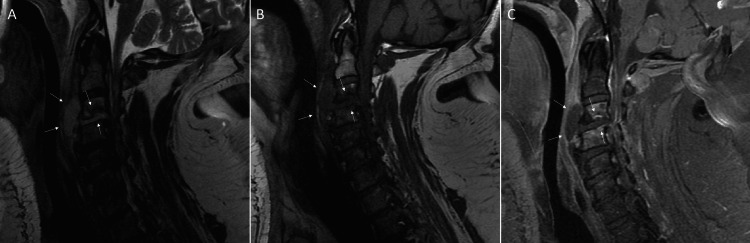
MRI of the cervical spine with (A) fat saturated T2 imaging, (B) T1 imaging, and (C) T1 imaging with gadolinium-based contrast showing evidence of discitis/osteomyelitis centered at C3-4 with adjacent paravertebral abscesses, and suggestion of epidural phlegmon in the anterior epidural soft tissues of C2 and C3.
